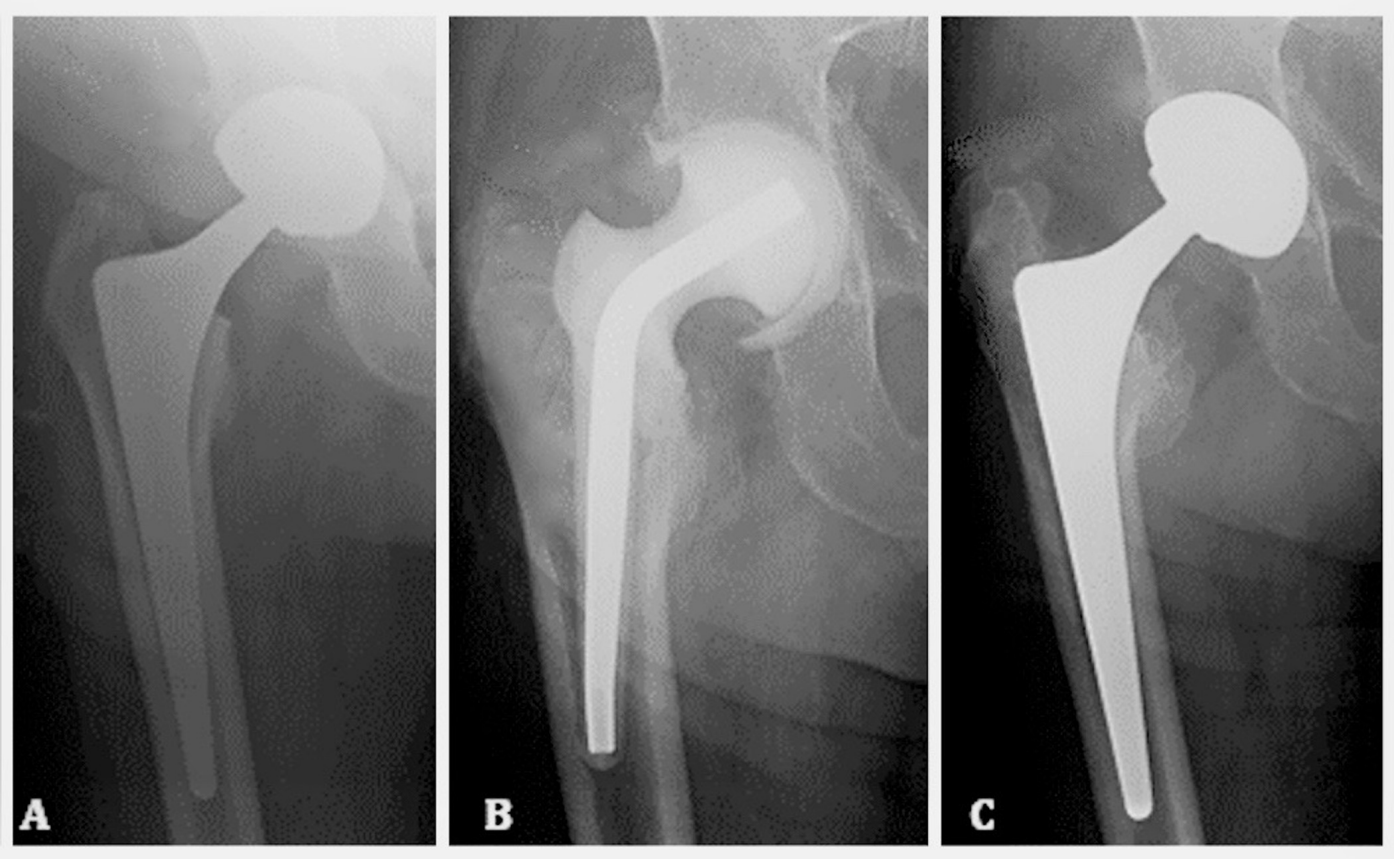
Radiographic example of two-stage revision for hip PJI with primary
